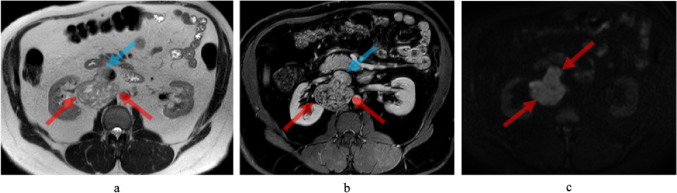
Axial T2-weighted image (a) without fat saturation and axial fat-saturated T1-weighted contrast enhanced image (b) demonstrate a heterogeneously enhancing right perirenal retroperitoneal mass with a large extravascular component (red arrows) and a small intravascular IVC component (blue arrows). Diffusion weighted image (c) shows high restriction diffusion within the mass (red arrows). Pathology confirmed leiomyosarcoma arising from the IVC
